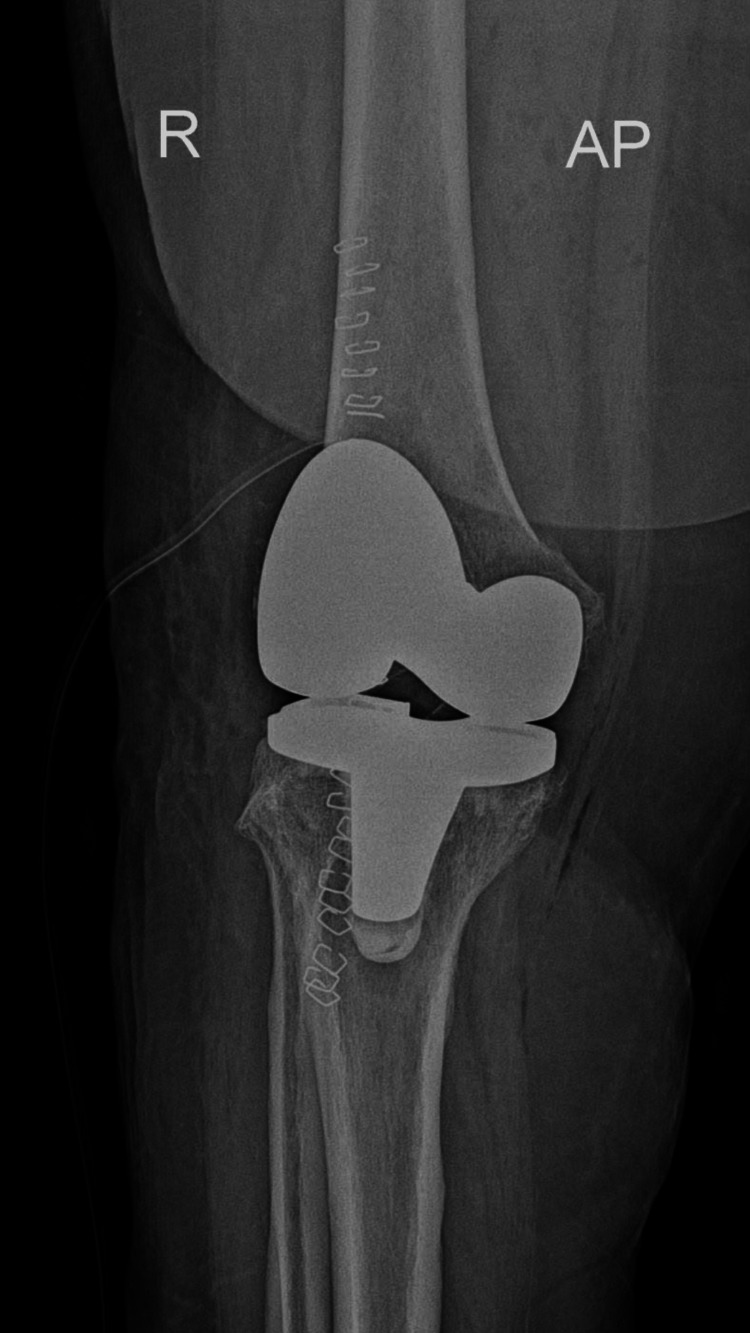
Immediate postoperative anteroposterior radiograph of the right knee Immediate postoperative anteroposterior radiograph of the right knee demonstrating satisfactory positioning and alignment of the femoral and tibial components following total knee arthroplasty.
